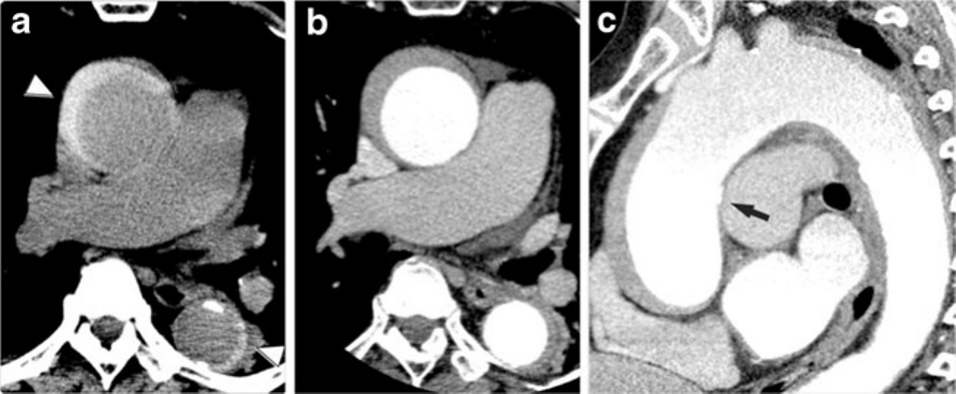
Dissection variant intramural haematoma (IMH) in a 54-year-old man who presented with acute chest pain. a Non-contrast CT image shows a crescent-shaped high-density area in the ascending aorta and descending aorta (*arrowhead*). b On the axial contrast-enhanced CT image, neither the flow channel nor intimal flap is detected along the entire aorta, consistent with IMH. c Oblique sagittal MPR image demonstrates widespread extension of type A IMH throughout the whole thoracic aorta. An ‘ulcer-like projection,’ the radiological finding of a focal disruption of the surface of the aortic wall from the true lumen into the thrombosed false lumen, is identified at the mid ascending aorta (*arrow*). During surgery, a small thrombosed focal primary intimal tear (PIT) was identified at the corresponding site
